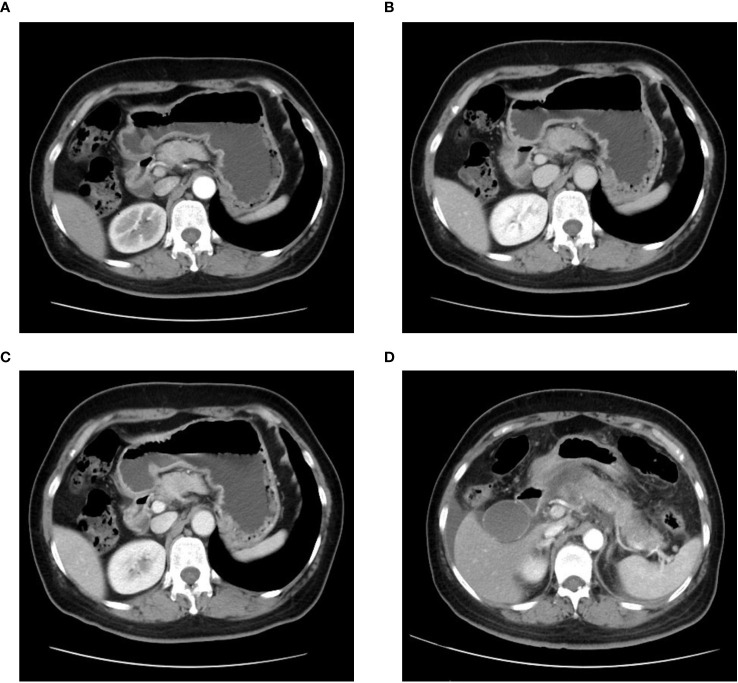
Computed tomography (CT) of the epigastric region showing a pancreatic mass with indeterminate nature is depicted in (A) arterial phase, (B) venous phase, and (C) delayed phase. (D) An enhanced CT image of the epigastric region taken during the patient’s initial episode of pancreatitis.
